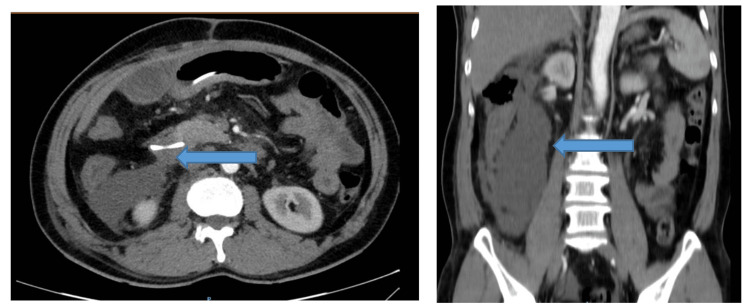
CT demonstrated a loss of continuity of the posterior duodenal wall at the second portion (D2), with a retroperitoneal fluid and gas collection extending to the right perirenal and paracolic spaces.
